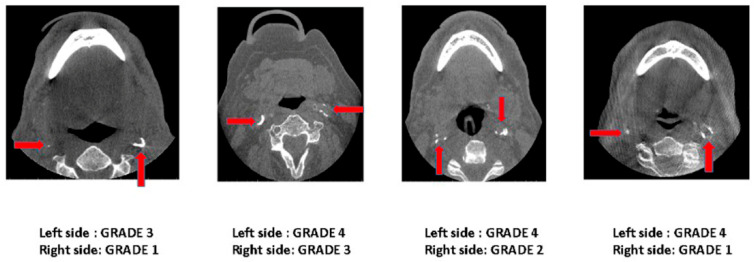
Axial-section CBCT images demonstrating bilateral ECACs according to the proposed grading scheme.
